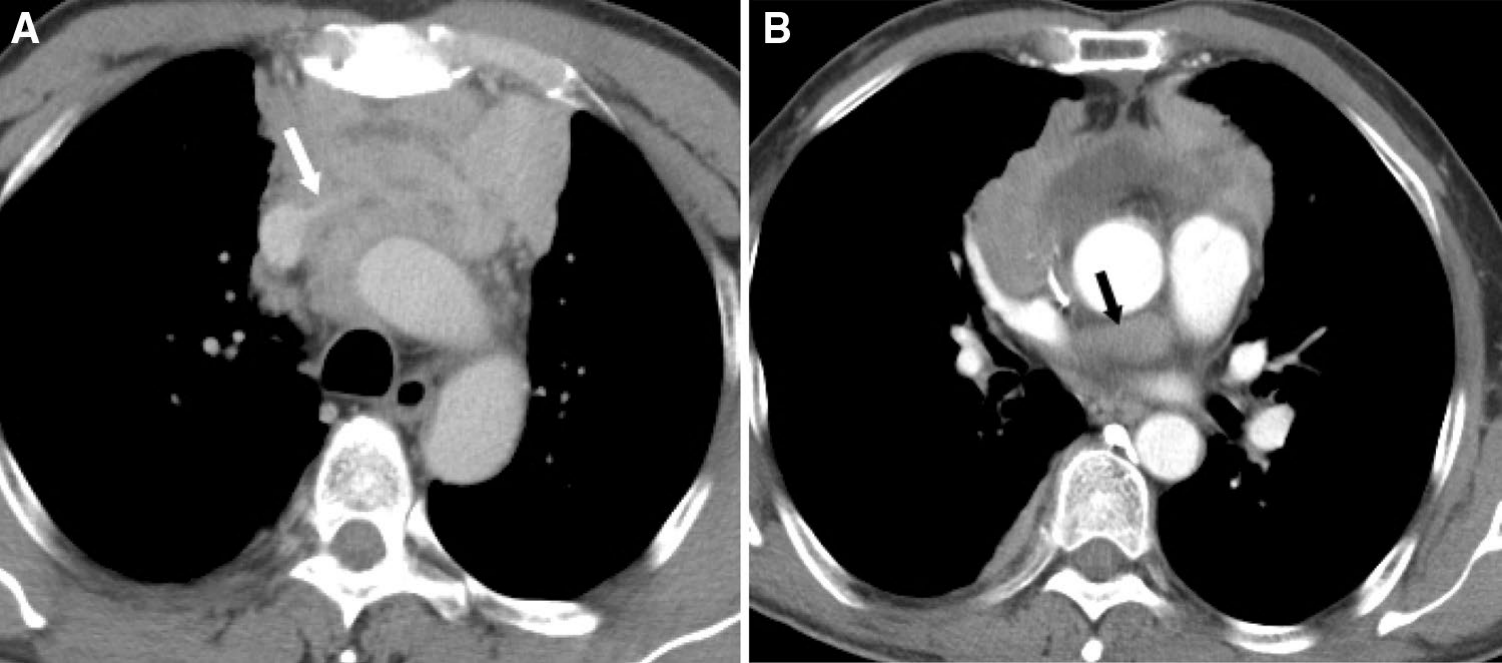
Thymic squamous carcinoma in a 54-year-old man with vessel invasion and pericardial mass. Contrast enhanced CT scan (Axial 5.0 mm) obtained at the level of the aortic arch (A) and pulmonary trunk (B) shows the interface between the tumor and the left brachiocephalic vein was blurred and the lumen was quite narrowed and compressed (white arrow). A soft tissue mass was seen inside and around the pericardium (black arrow). This patient had tumor progression and died 43.9 months since the first CT examination.
